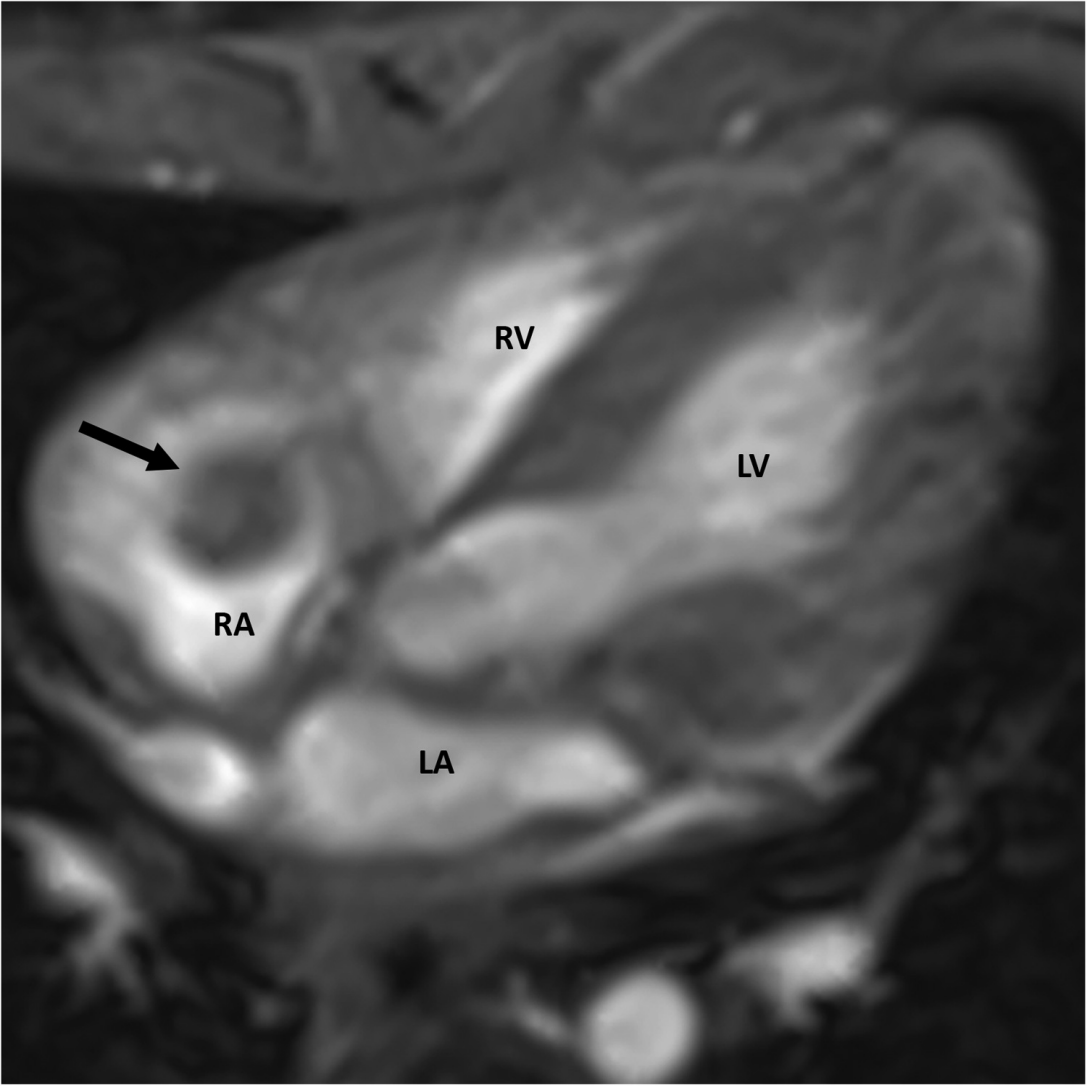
Cardiac MRI showing a well-defined mass (arrow) within the right atrium. RA, right atrium; LA, left atrium; RV, right ventricle; LV, left ventricle.
